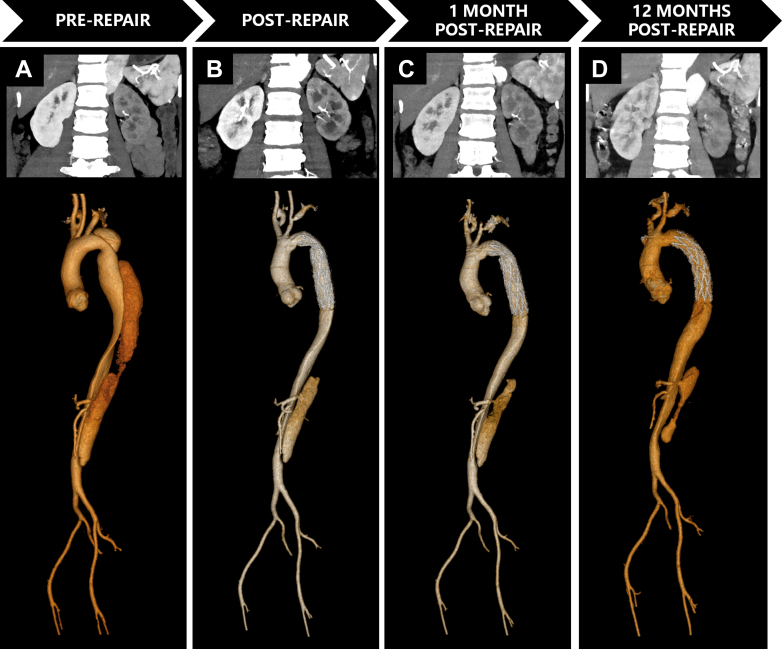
Serial Computed Tomography Angiography Demonstrating Aortic Evolution and Progressive Renal Atrophy in Patient 2 (A) Preoperative: Stanford type B AD involved renal arteries. LRA originates from FL, RRA from TL with left renal hypoperfusion despite symmetric volumes. (B) Immediately postsurgery: Persistent hypoperfusion of the left kidney. (C) One-Month CTA: FL thrombosis with mild volume reduction. Left renal hypoperfusion persists, but volume remains preserved. (D) Twelve-Month CTA: Complete FL thrombosis extending to LRA origin causing critical inflow obstruction. The left kidney volume lost (18% preoperative volume) and cortical thinning. The right kidney showed compensatory hypertrophy.
